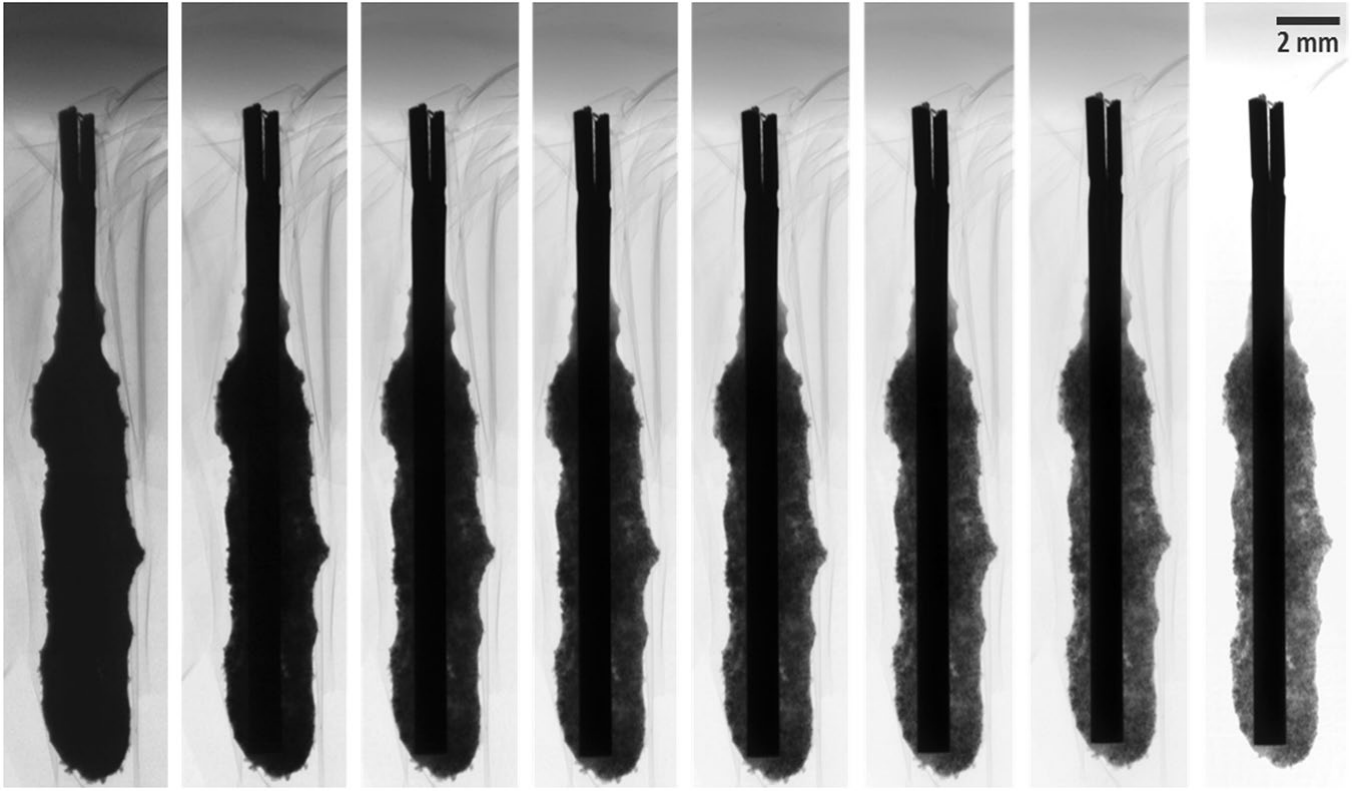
Radiography of a deposited sample obtained by 10,000 second by CP with a constant current of −7 mA at various voltages, 20, 30, 40, 50, 60, 80, 100, and 120 kVp at a constant current-exposure time product of 8.8 mAs (from the left). The darkest rod in each image is the tungsten electrode. Display window is set to be [0, 16384] a.u. (Supplementary Video S1).
